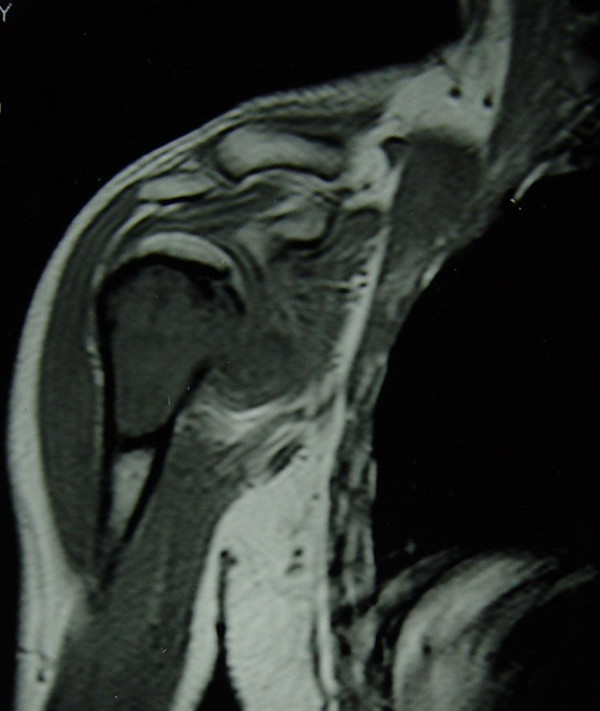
Preoperative MRI image of the same patient.
